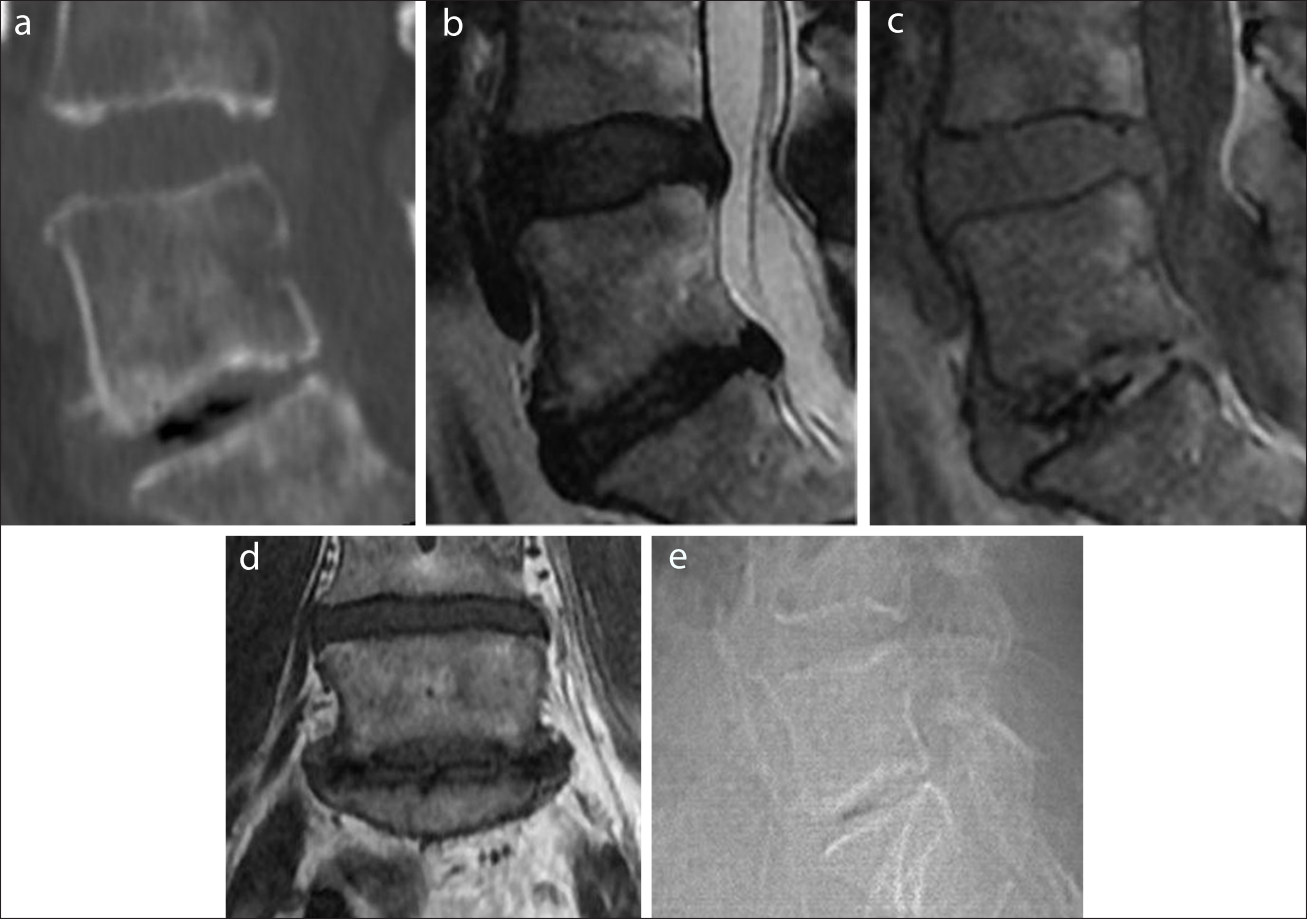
VP as intradiscal gas in the lower (L5-S1) of the two visualized discs (L4-L5 and L5-S1) on a sagittal reconstruction of a CT lumbar spine in bone window (a), a sagittal T2- (b) and GE-weighted image (c), a coronal T1-weighted image (d), and the lateral scout image from the CT exam (e).
